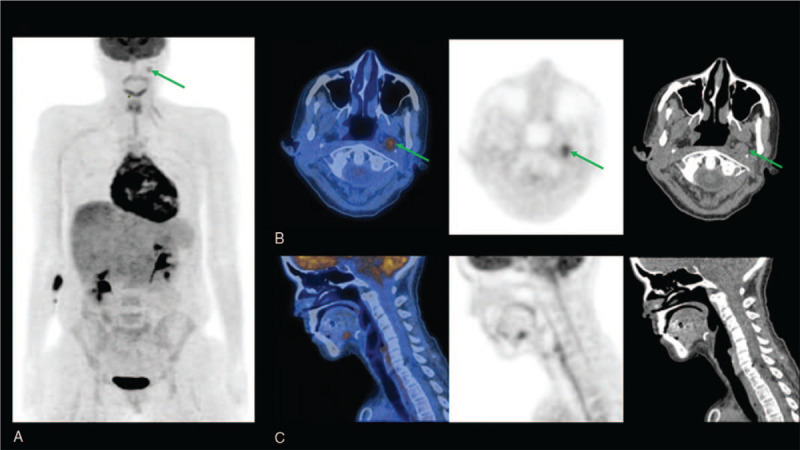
(A) Anterior view of 3D Maximum intensity projection PET image showing an intense and pathological FDG uptake in the basal posterolateral wall of the right ventricle and in the pericardium with a low FDG uptake in one left retropharyngeal lymph node consistent with metastasis (green arrow). (B) Axial PET/CT, PET, and CT images of the nasopharynx showing the pathological left retropharyngeal lymph node (green arrow). (C) Sagittal PET/CT, PET, and CT images showing no tongue abnormality.
